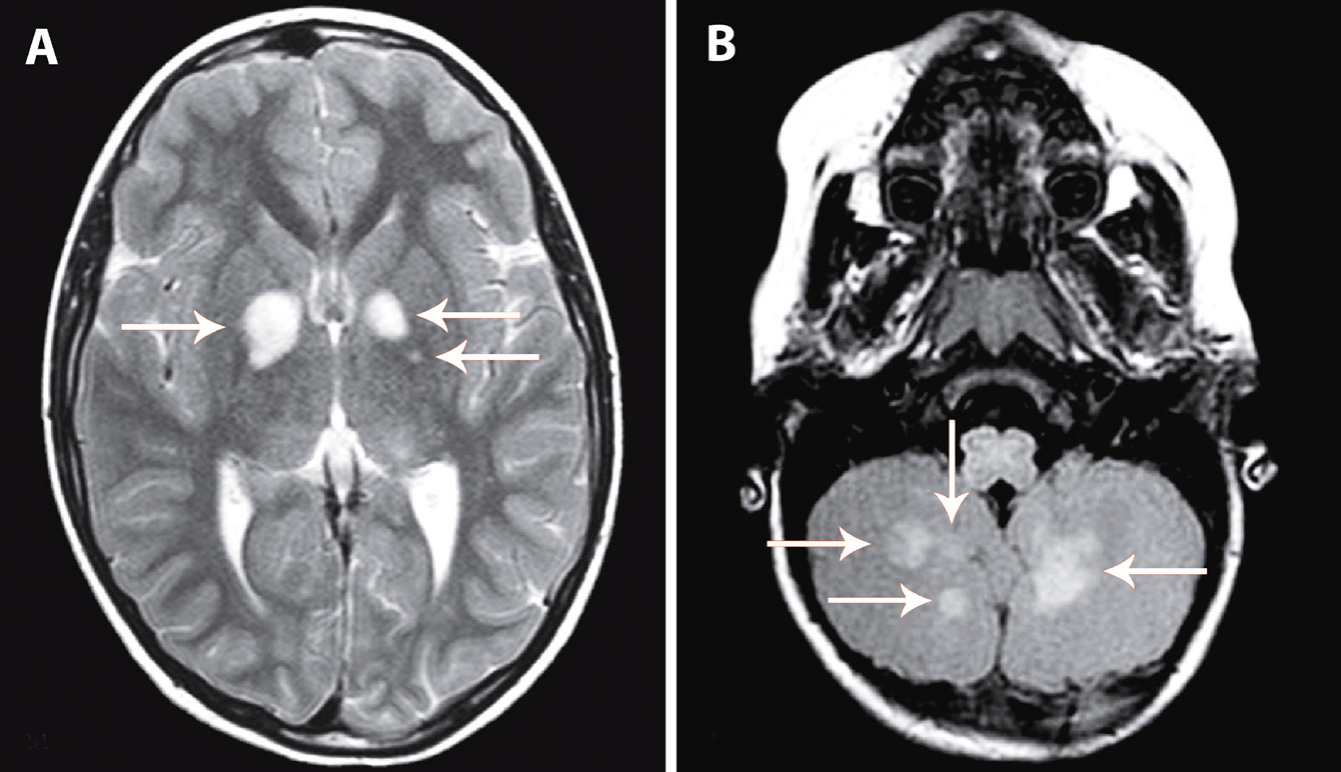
Multiple hyperintensities on T2-weighted cranial magnetic resonance imaging in children with neurofibromatosis type 1. Axial image shows multiple oval discrete lesions with increased intensity (arrows) in the globus pallidus (A); axial fluid attenuated inversion recovery image shows multiple diffuse T2-hyperintensities (arrows) in the cerebellum (B).
